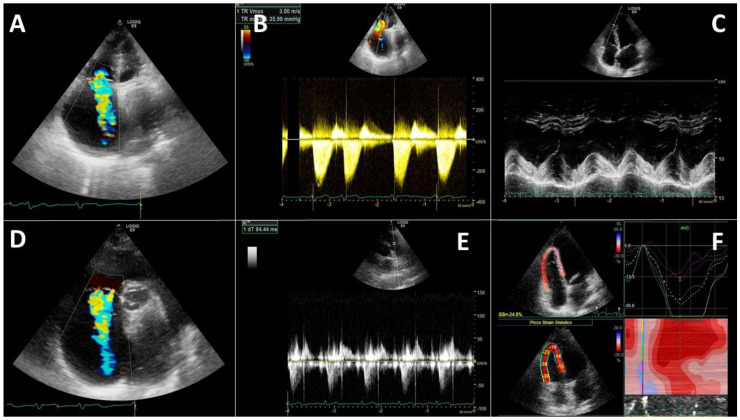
Right heart evaluation needs an apical 4 chamber view (A) useful to detect the tricuspid regurgitation velocity (B) and the tricuspid annular plane excursion (TAPSE) (C). The parasternal short-axis view (D) allows another possible evaluation of tricuspid regurgitation as well as the pulmonary artery analysis (E). A right ventricle longitudinal strain (F) is possible in advanced imaging from an apical 4 chamber view.
